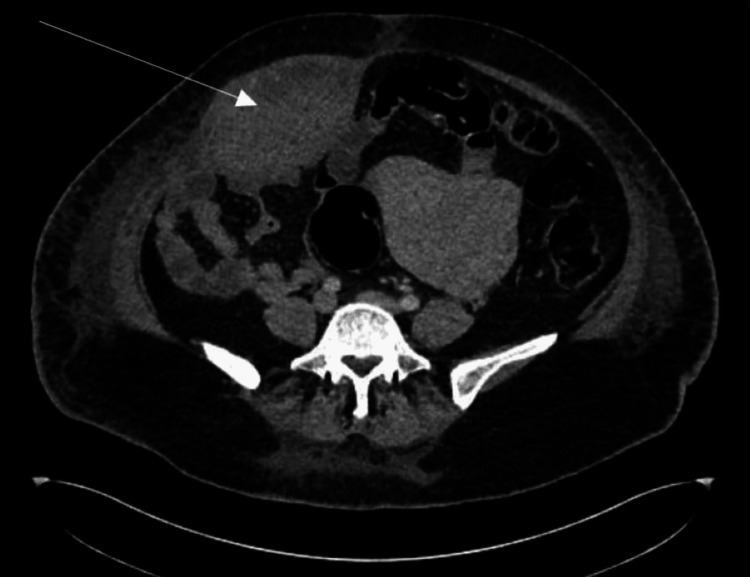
Computed tomography (CT) scan image of the rectus sheath haematoma, coronal view (arrow indicates the haematoma).
